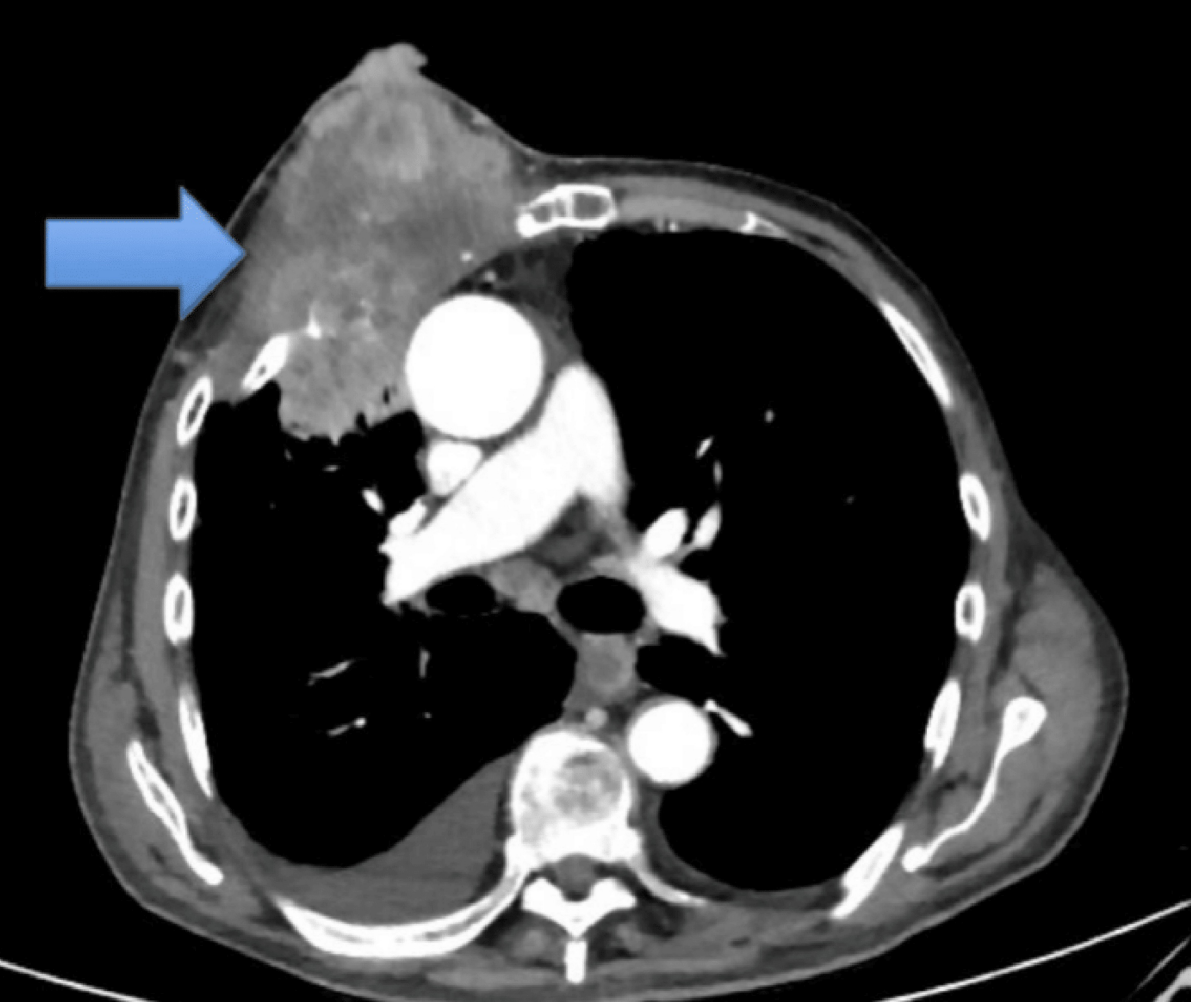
Contrast-enhanced computed tomography (CT) image showing right chest wall mass measuring 11x6 cm invading the third and fourth rib and extending superficially towards the skin
